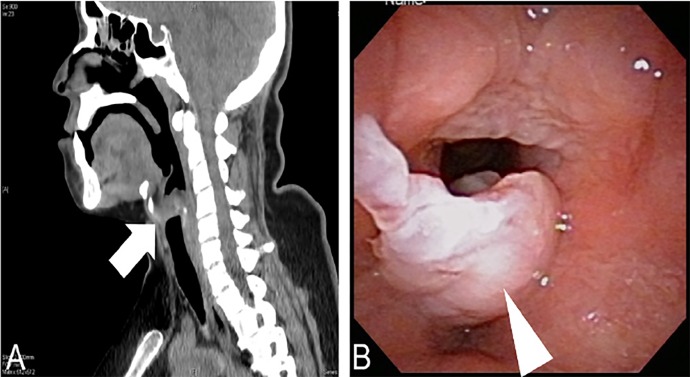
A) Sagittal cervical computed tomography showing a hypodense mass below the epiglottis (arrow).B) Laryngoscopy showing an elevated vocal polyp (arrowhead).
